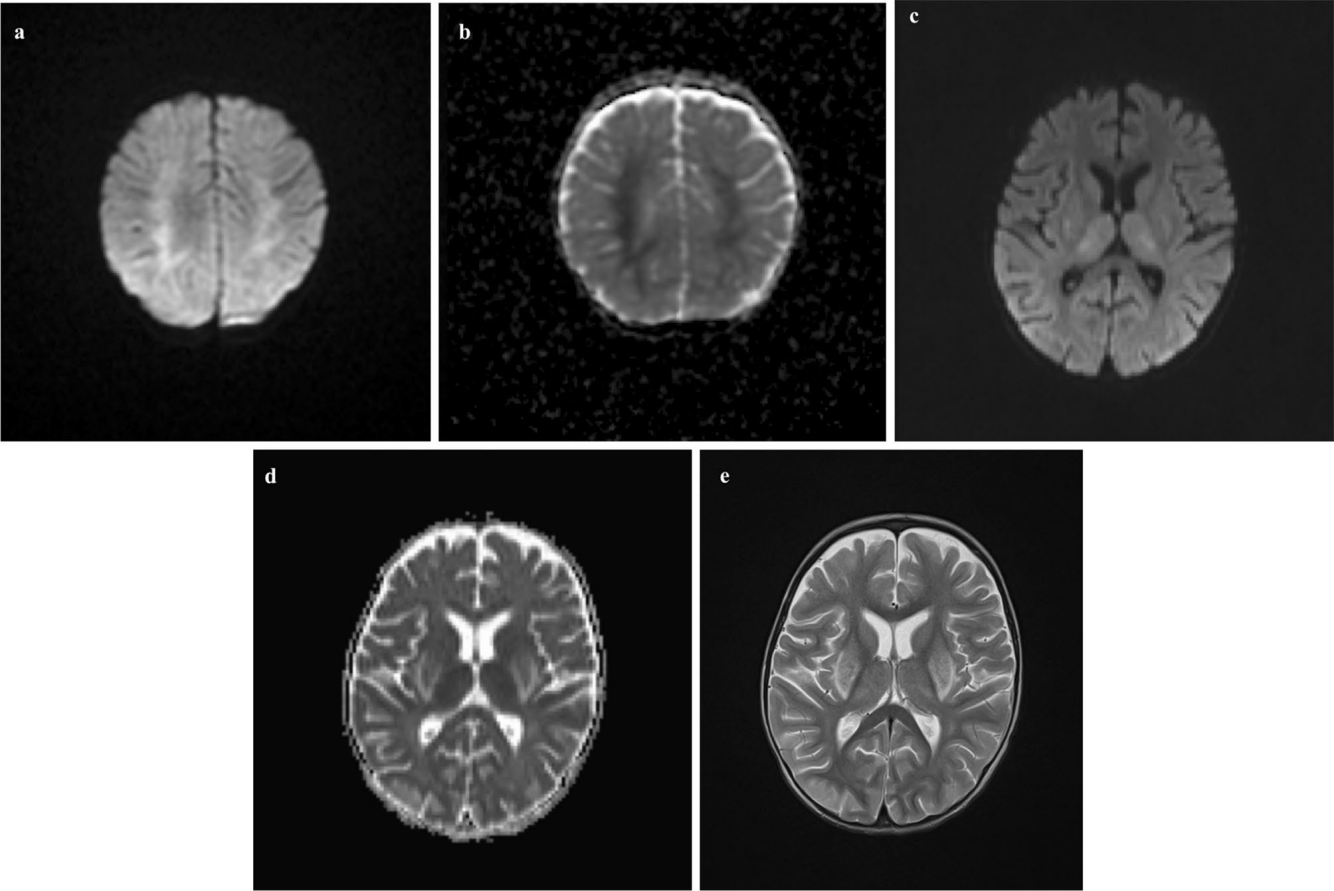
a, b Patient 1: axial DWI (a) and ADC map (b) show reduced diffusivity in both centrum semi-ovale which extended inferiorly to the periventricular white matter adjacent to bilateral frontal horns. c–e Patient 16: axial DWI (c) and ADC map (d) show restricted diffusion in the thalami bilaterally with increased diffusion within the periphery of the lentiform nuclei. There is corresponding increased signal abnormality in grey matter structures on axial T2 images (e)
